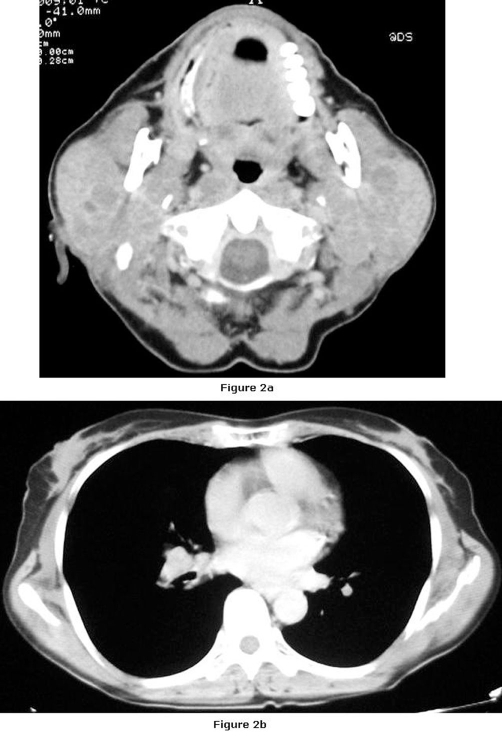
(A) Cervical CT of the patient before the treatment. (B) Thorax CT of the patient before the treatment.
